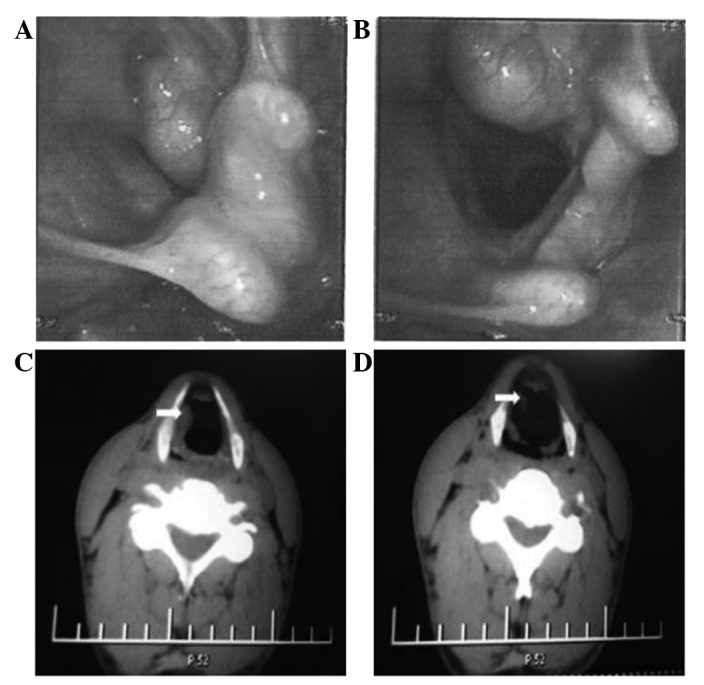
(A and B) Electronic laryngoscopy revealing a submucosal mass in the right supraglottic area; laryngeal morphology and motility were preserved. (C and D) Axial computed tomography scan showing an 8×11-mm expansile soft-tissue mass in the right supraglottic area, with no enlargement of the lymph nodes in the neck.
